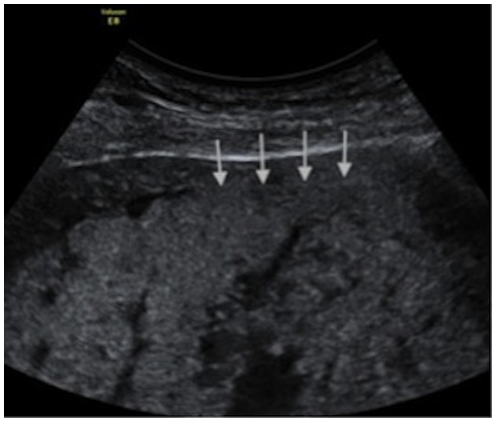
Loss of the normal retroplacental clear space on ultrasonography.
